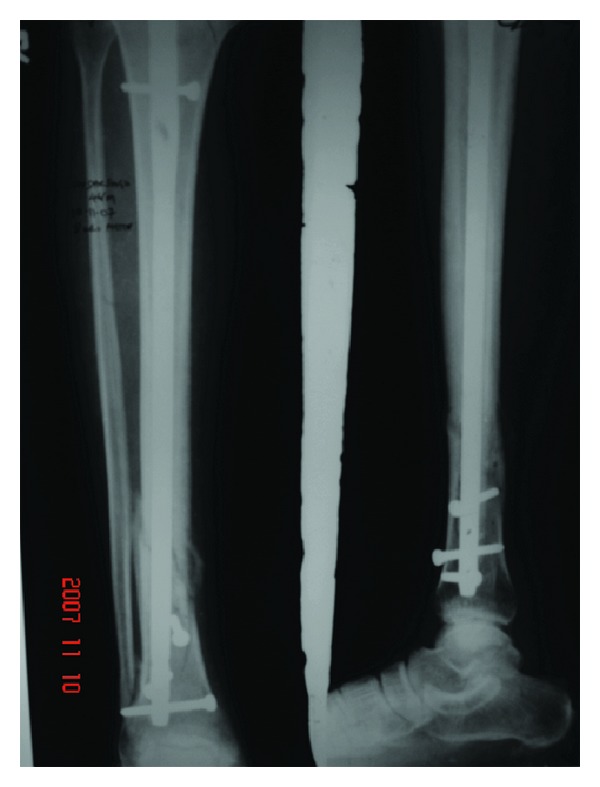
Eight-week postoperative X-ray showing fracture union without any loss of reduction.
